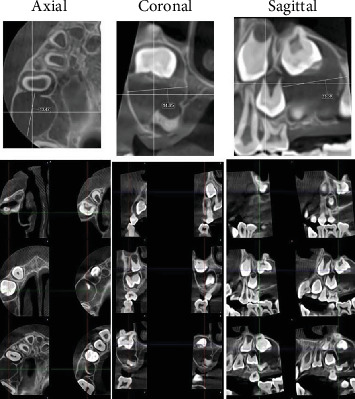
Axial, coronal, and sagittal CBCT images showing a well-defined unilocular radiolucency in the right posterior maxilla associated with the unerupted upper right first molar.
